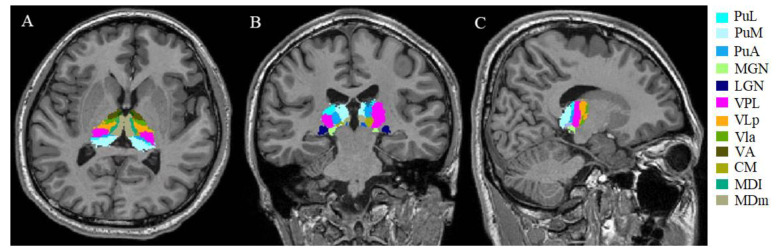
Example of segmentation of thalamic nuclei. Segmentation and labeling of thalamic nuclei in axial plane (A), coronal plane (B), and sagittal plane (C) generated by FreeSurfer (not all segmentations are shown). The segmentations are overlaid on the 3D T1-weighted scan. PuL: pulvinar lateral, PuM: pulvinar medial, PuA: pulvinar anterior, MGN: medial geniculate, LGN: lateral geniculate, VPL: ventral posterolateral, VLp ventral lateral posterior, VLa: ventral lateral anterior, VA: ventral anterior, CM: centromedian, MDl: mediodorsal lateral parvocellular, MDm: mediodorsal medial magnocellular nucleus.
